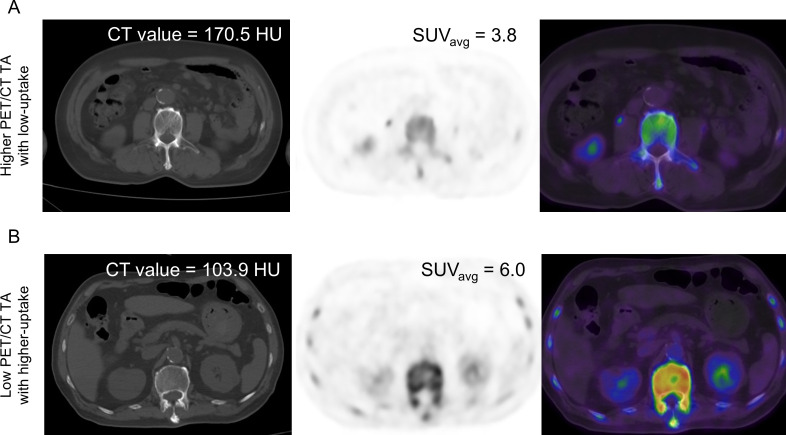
Example of metabolic-structural discordance. (A) Higher PET/CT TA but low-uptake phenotype. (B) Low PET/CT TA but higher-uptake phenotype.
